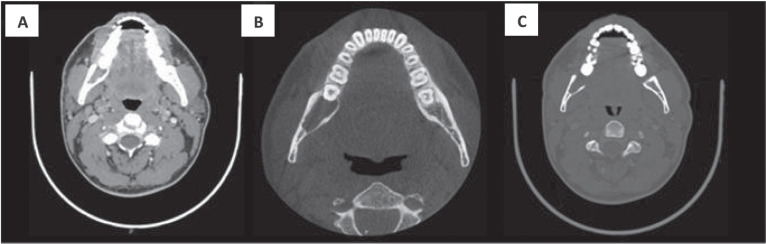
CT with intravenous contrast. Both the soft tissue window (a) and bone windows (b–c), shows an osteolytic lesion with right mandibular body and ramus involvement, localised in the retromolar space behind tooth number 48. The lesion features soft tissue components and presents lingual cortical disruption. It shows the involvement of the adjacent inferior alveolar nerve canal. It measures approximately 29 × 23 × 11 mm in size.
